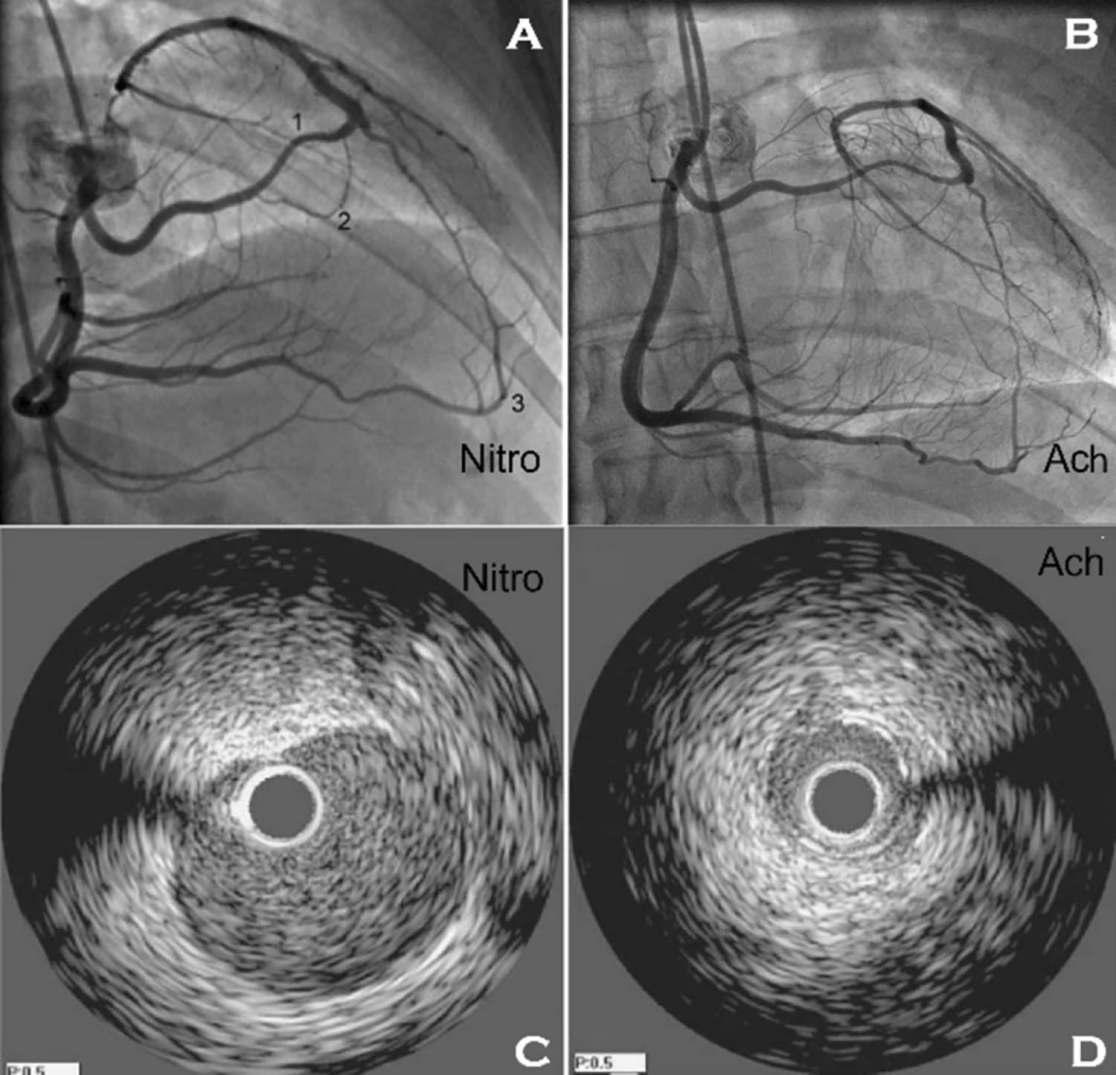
L-ACAOS-PP: inducible spasm of the left main coronary artery. A 32-year-old man with a long-term history of severe, prolonged resting angina, occasional mild troponin elevation, and normal left ventricular function presented to the emergency department. Treadmill testing was negative for angina and ST-T changes. A An angiogram after nitroglycerin administration shows homo-collateral at left main artery (site 2) and periapical collateral from the right coronary artery (site 3), but no spasm (site 1). B An angiogram at positive ACh testing. Intravascular ultrasonography shows C a dilated left main segment after nitroglycerin and D severe, subtotal spastic stenosis upon redo ACh challenge (new stenosis at site A1). Spasm was less severe at B than at D, where mechanical stimulation from the IVUS catheter (site A1) induced 90% stenosis (versus 60%). Severe Prinzmetal angina was diagnosed and responded well to vasodilators, improving from NYHA classification III-IV to II. Calcium antagonists (nifedipine 60 mg/day) and sublingual nitroglycerin provided fair palliation for 11 years, but worsening angina with syncopal spells eventually led the patient to opt for vein-graft surgery to the left anterior descending coronary artery, which adequately resolved symptoms. Eight years later, his nuclear PET scans were negative for reversible ischemia or scar, and left ventricular function was maintained within normal limits. *ACh* indicates acetylcholine, *L-ACAOS-PP* anomalous origin of the left coronary artery from the right sinus of Valsalva, with pre-pulmonic course, *Nitro* nitroglycerin. Source: Sanford GB, Molavi B, Sinha AK, Garza L, Angelini P. Single coronary artery with prepulmonic coursing left main coronary artery manifesting as Prinzmetal’s angina. *Tex Heart Inst J.* 2007;34(4):449–452
